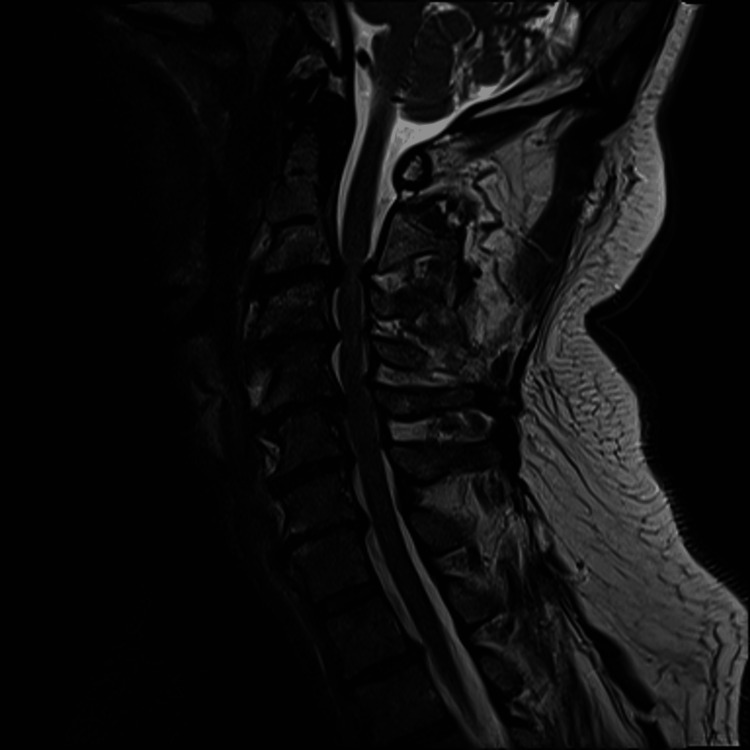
Sagittal T2-weighted MRI of the cervical spine.
